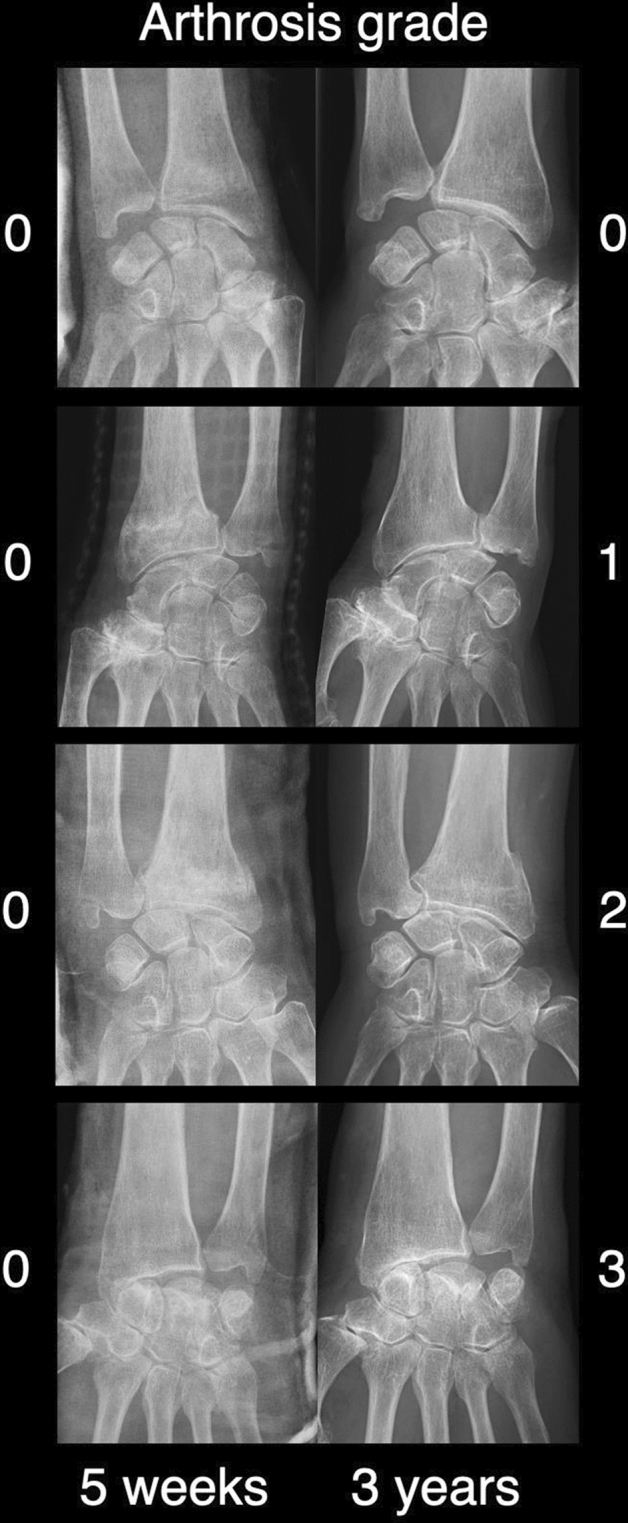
Examples of assessed anteroposterior radiographs with posttraumatic arthritis (PA) grades 0, 1, 2, and 3 after 5 weeks and a mean of 3.3 years after the injury.
